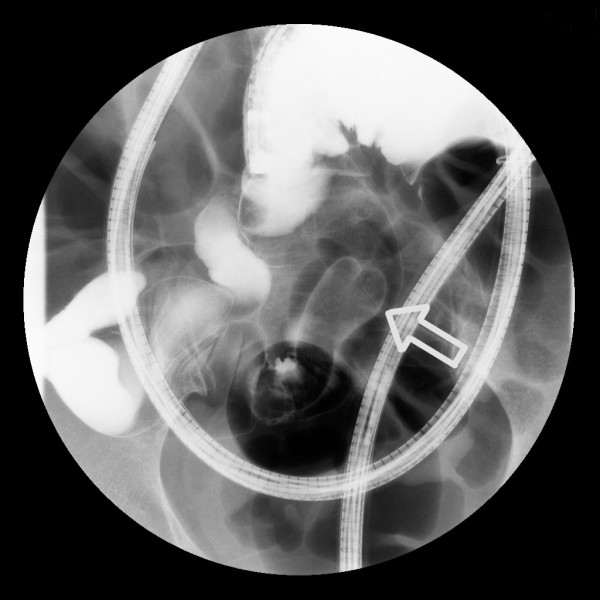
Selective contrast-enhanced radiography. A dumbbell-shaped tumor was shown by selective contrast-enhanced radiography.
